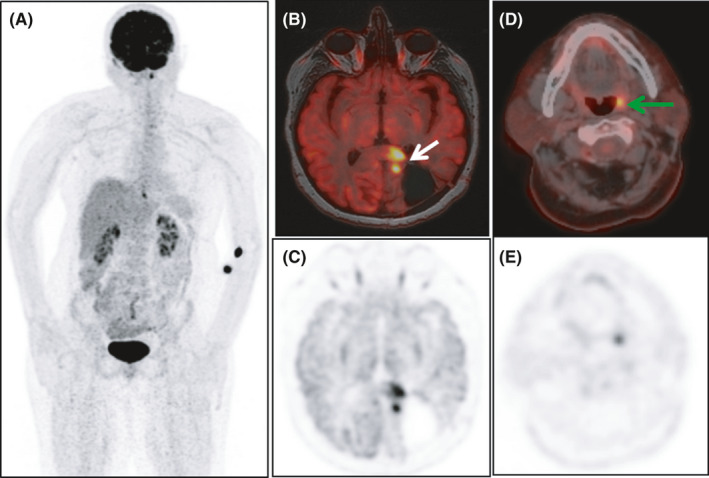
To examine the spread of disease, whole‐body 18F‐FDG PET/CT and brain PET/MRI were performed. The study revealed hypermetabolic lesions in the left occipital lobe, left tonsil, and distal esophagus in maximum intensity projection (MIP) images (A). 18F‐FDG PET/MRI images showed multiple intense hypermetabolic lesions (SUVmax: 22.75) adjacent to the operative defective area extending from the left occipital lobe level to the parietal lobe in the fused axial PET/MRI (B, C—solid arrow). Asymmetrical uptake of FDG (SUVmax: 9.68) demonstrated by the arrow on the axial 18F‐FDG PET/CT images localizes to the left tonsil (D, E—solid arrow)
